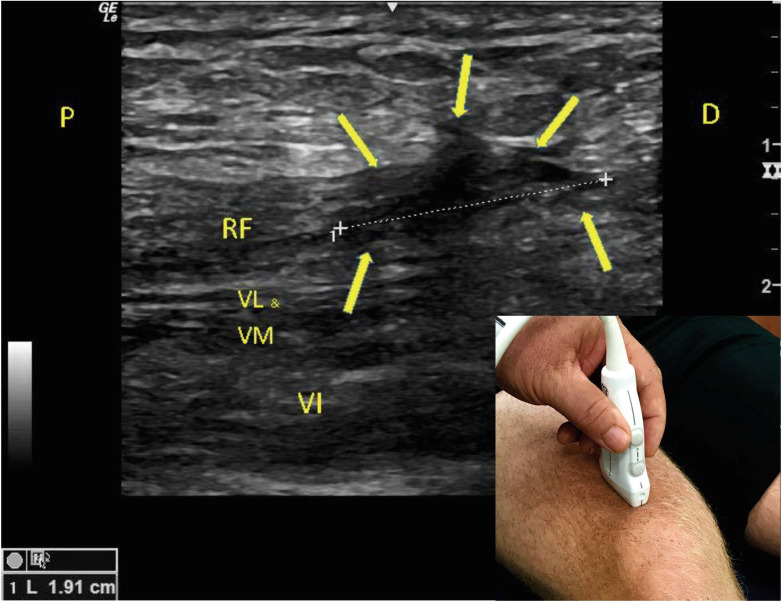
Post-injurylong-axis ultrasound image of the Rectus Femoris (RF) (5-12 MHz linear transducer). The proximal and distal aspect of the rectus femoris exhibits a normal hyperechoic appearance, but a substantial hypoechoic area is noted (arrowheads), 1.9 cm which is suggestive of fluid and a full thickness mid-tendon tear of the rectus femoris. Also, mild hypoechoic signaling indicating fluid in the medial and lateral muscles indicating a strain with mild hypoechoic signaling deep indicating strain of vastus intermedius. Positioning of the ultrasound probe is seen in the bottom right-hand corner of the image. Abbreviations: P: proximal; D: distal; RF: rectus femoris; VL: vastus lateralis; VM: vastus medialis; VI vastus intermedius.
